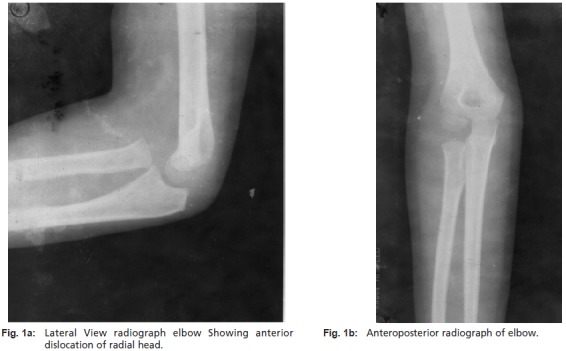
(1a)Lateral View radiograph elbow Showing anterior
